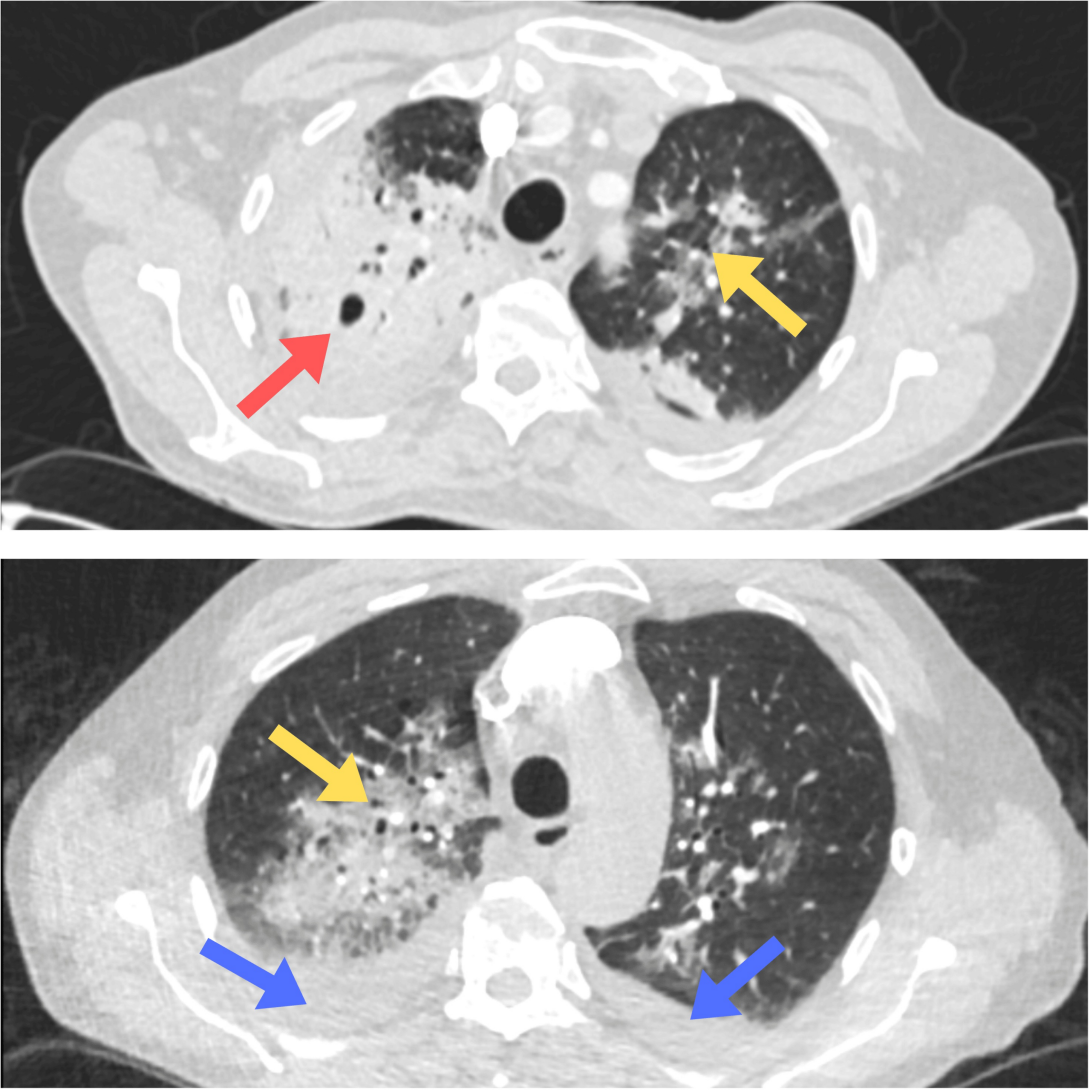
CT Thorax showing multifocal bilateral pneumonic infiltrates, bilateral pleural effusions, and organizing pneumonia Red arrow showing cavitatory lesion in right lung, yellow arrow showing consolidation. Blue arrows showing bilateral pleural effusions.
